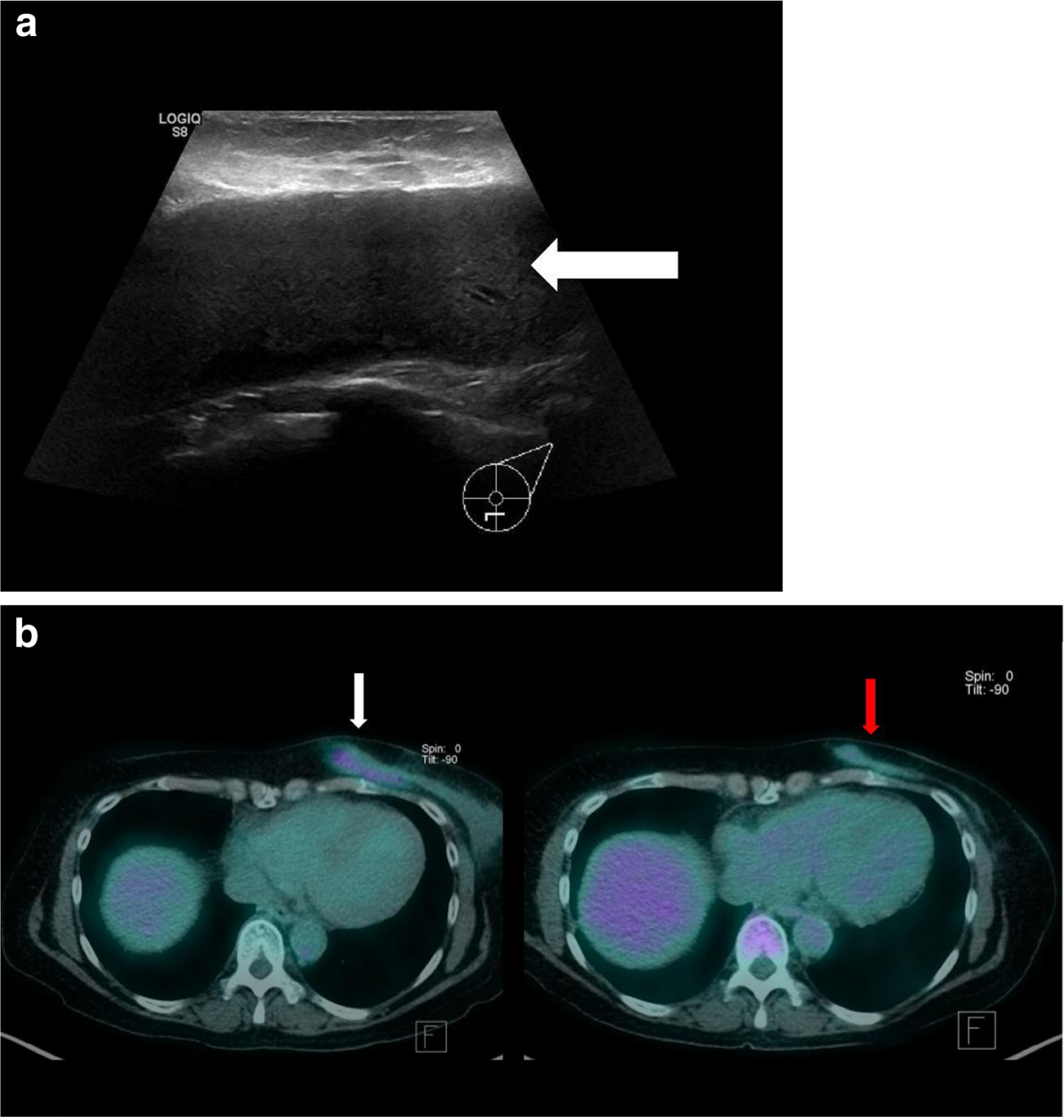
a Ultrasound revealed a large effusion with no signs of infection (*white arrow*). Fortunately, the aspirated fluid was sent for cytology, which confirmed BIA-ALCL. b PET/CT revealed a flattened rim of soft tissue, located inferomedially in the left breast, with ill-defined margins and moderate FDG uptake (*white arrow*). The patient subsequently received six cycles of chemotherapy and targeted radiotherapy. Restaging PET/CT revealed complete metabolic response (*red arrow*)
